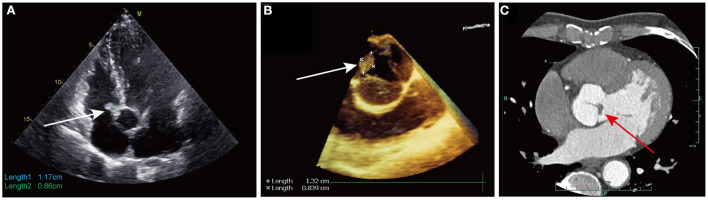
(A) Transthoracic echocardiography showed an irregular hyperechoic mobile mass (1.2 × 0.9 cm in size, white arrow) with a stalk arising from the right atrium adjacent to the aortic root; (B) Transesophageal echocardiography showed an additional mobile mass (1.3 × 0.8 cm in size, white arrow) on the ventricular side of the non-coronary cusp of aortic valve; (C) Coronary computed tomography angiography showed abnormal thickness of non-coronary cusp of aortic valve, which represented the cardiac papillary fibroelastoma (red arrow) on the aortic valve.
